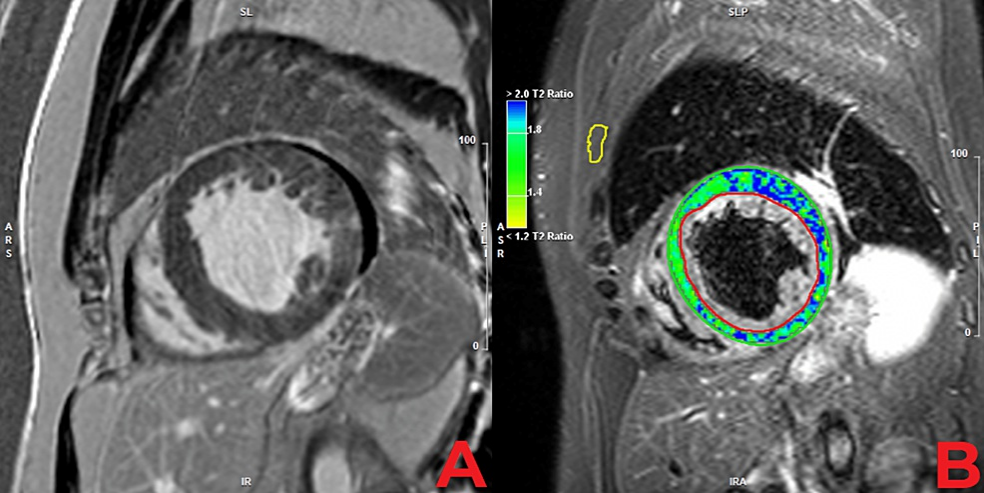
Cardiac magnetic resonance imaging showing a small area of hazy, subepicardial late gadolinium enhancement in the mid-inferolateral segment, adjacent to a pocket of effusion (Panel A) with concurrent elevated signal intensity on T2-weighted short tau inversion recovery images (Panel B), suggestive of mild myocarditis with active inflammation. There is also evidence of prominent left ventricular trabeculations.
